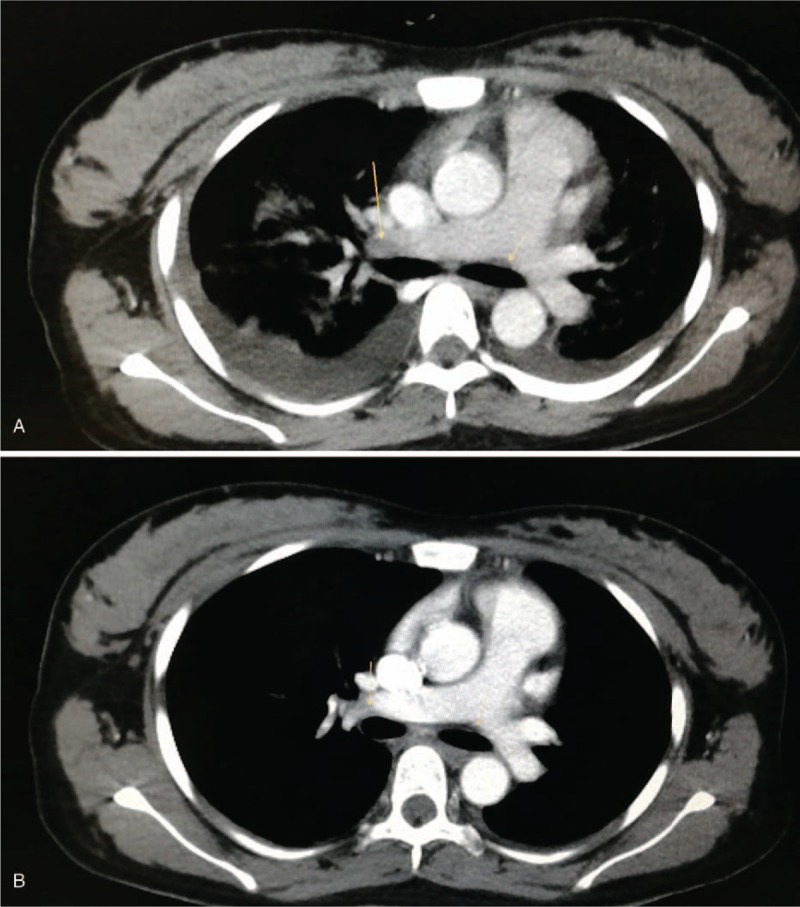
Contrast-enhanced CT scan revealed hypodense filling defects in pulmonary arteries (A) and re-examination showed reduction of the hypodense filling defects (B) after anticoagulant therapy. CT = computed tomography.
